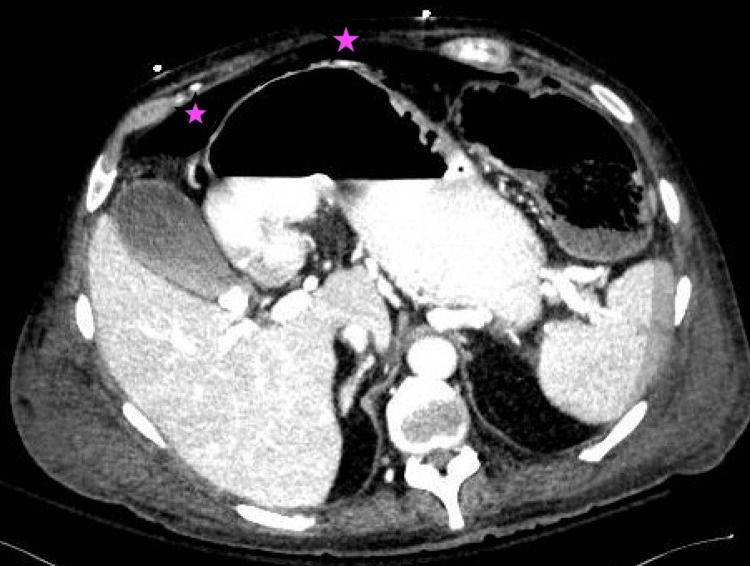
CT abdominal and pelvis. A large amount of pneumoperitoneum (marked star) without an exact free air source.
